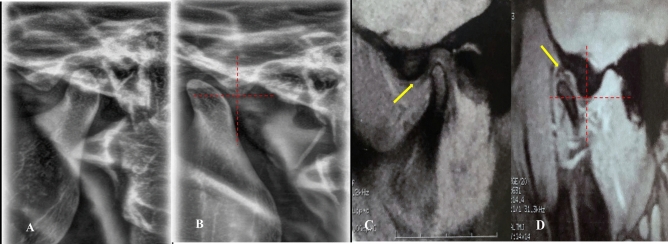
Lateral TMJ view represents stage II-A in closed (A) mouth position and in open (B) mouth position, showing the condyle located anterior to the eminence but at a superior level to the highest point of the eminence. Oblique sagittal T1-weighted images represent Stage II-A, showing a normal condyle-disk-fossa relationship (yellow arrow) in closed (C) mouth position and in open (D) mouth position, with the disk-condyle complex located anterior to the eminence but high up in front of the base of the eminence.
